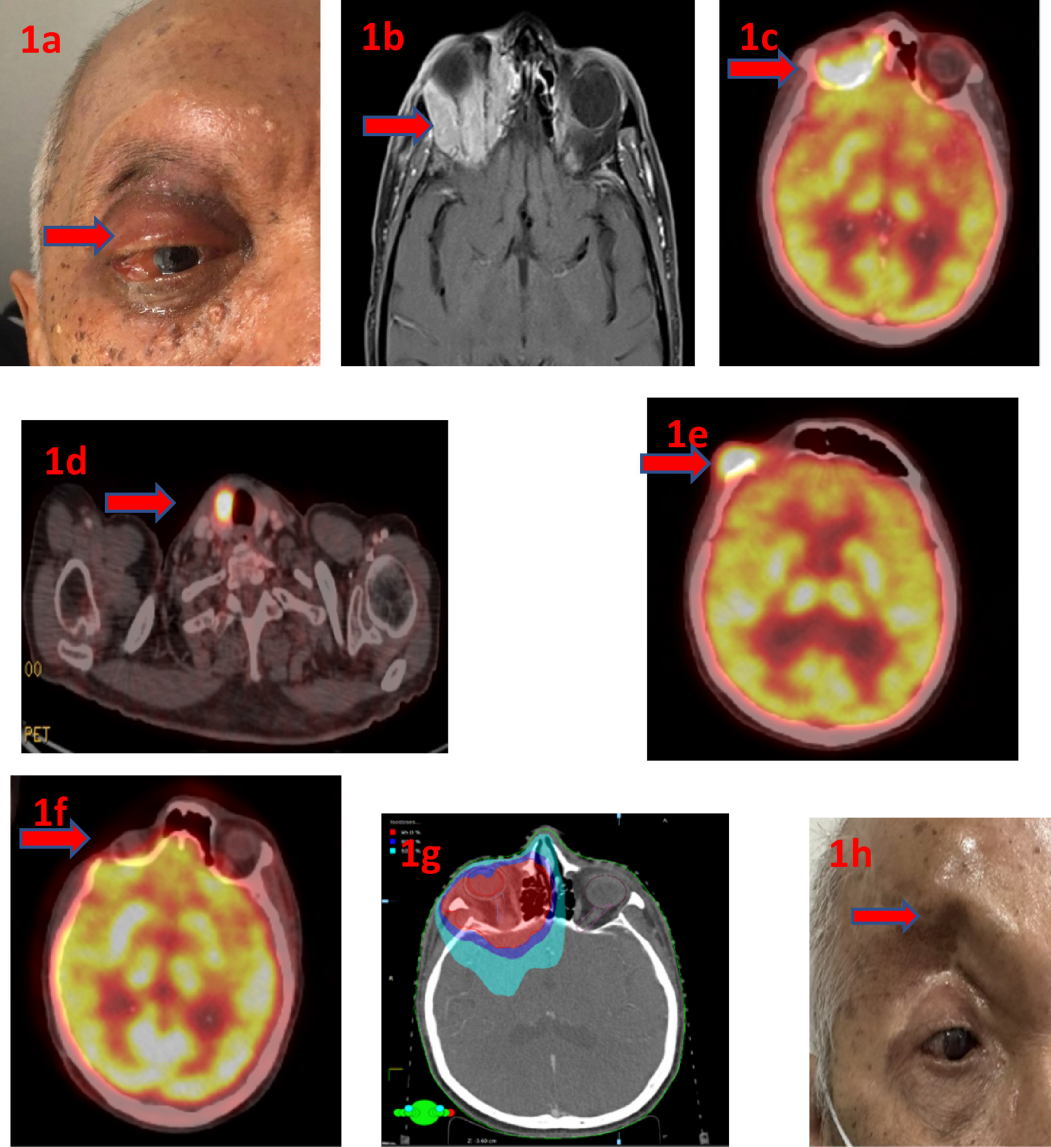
(a) Patient photograph during treatment, showing edematous conjunctiva and swelling. (b) Arrow marked showing hyperintense (T1 contrast of MRI orbit after post‐excision biopsy) soft tissue mass involved the retro‐orbital right side area with extension to medial and lateral aspects. (c and d) Arrow marked region showing the FDG avid (PET‐CT images before treatment) area in the right side of the orbit (c) with FDG avid region in the right lobe of thyroid (d). (e) After CCRT and before reconstructive surgery, arrow mark area showing high FDG avid region of residual disease. (f) Arrow marked area showing no regional FDG uptake (follow‐up PET scan after 2.5 years of treatment) in the primary right orbit. (g) Rapid arc dose color wash during radiotherapy showing coverage in PTV, 95% in red, 80% in blue, and 50% in turquoise color. (h) Recent photograph during last follow‐up visit in July 2023, 3.3 years after treatment.
